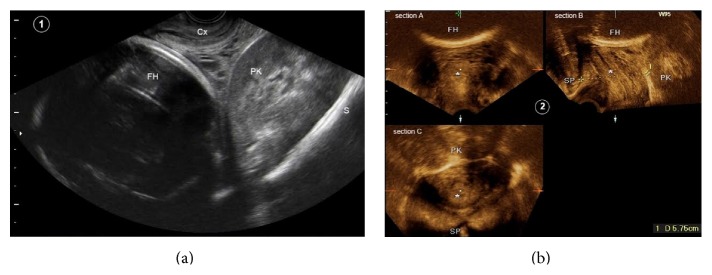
Transvaginal sonograms performed before cesarean section. (a) shows a sagittal B-mode sonogram of the maternal pelvis. The pelvic kidney (PK) is located between the sacral wall (S) and uterine cervix (Cx). The fetal head (FH) is positioned above the kidney and cervix. A three-dimensional multiplanar reformatted sonogram of the maternal pelvis is demonstrated in (b). The 3D volume was obtained by the sagittal acquisition using Voluson E8 ultrasound machine (GE Healthcare) and transvaginal probe (RIC 5-9H/OB). The maternal pelvis is orientated in three orthogonal planes: the coronal plane in section A; the median plane (section B) showing the cervix (*∗*) and fetal head (FH); and the axial plane in section C. Symphysis pubis (SP) and pelvic kidney (PK) appear in the left and right sides of section B, correspondingly. The internal edge of SP and the anterior margin of PK are indicated by the white arrowheads and small white arrows, respectively. The anteroposterior diameter of the pelvic canal between SP and PK was only 5.75 cm. Similarly, in the axial plane (section C) the pelvic canal shows a deformed shape due to the bulging of the pelvic kidney.
